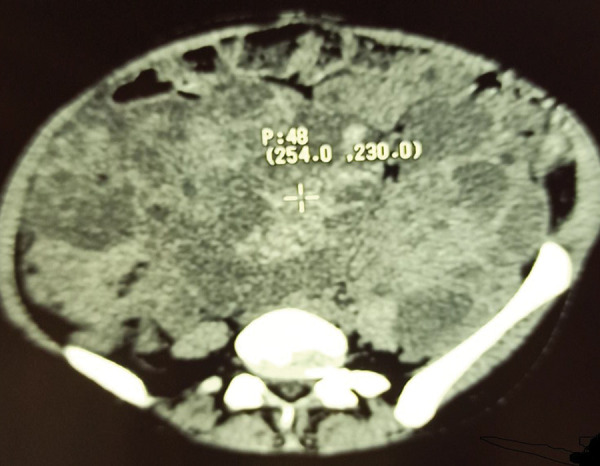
Axial CT-scan showed spontaneous intracystic hyperdensities (70 HU) corresponding to hemorrhages.
